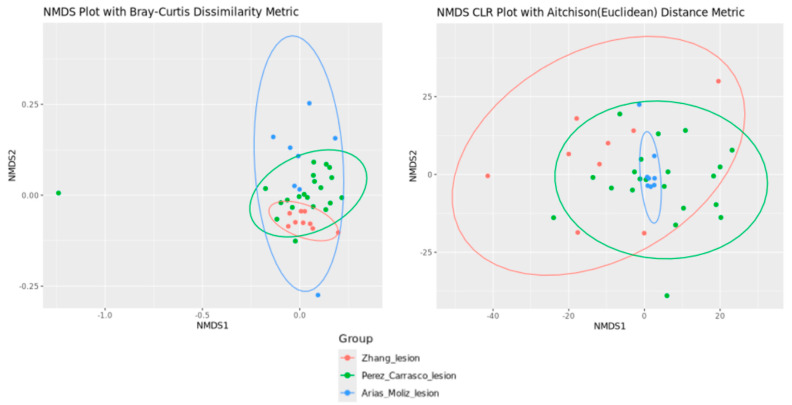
Beta diversity analysis of the microbiota in periapical lesions of the three included studies using non-metric multi-dimensional scaling (NMDS) with the Bray—Curtis dissimilarity metric or Aitchison distance metric.
